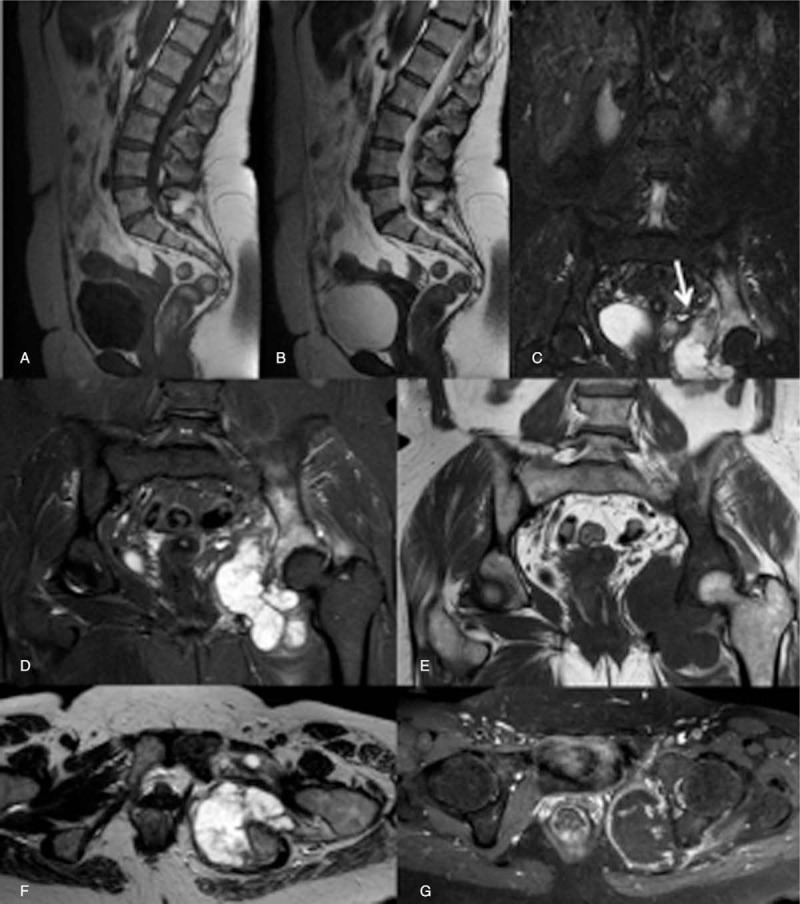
A 52-year-old female patient with chronic low back pain. (A) Sagittal T1-weighted sequence. (B) Sagittal T2-weighted sequence. (C and D) Coronal-STIR sequence. (E) Coronal T2-weighed sequence. (F) Axial T2-weighted sequence. (G) Axial T1-weighted 3D fat-sat MR sequence after contrast agent injection. Conventional MR sequences acquired on sagittal plane showed the presence of a mild disc extrusion at the level of the L5–S1 intersomatic space and a disc protrusion at the level of the L2–L3 intersomatic space (A and B). On coronal-STIR (C), a large expansive mass of the ischiopubic ramus with diffuse signal hyperintensity of the iliac bone was appreciated (arrow). The mass would have been missed on sagittal plane. (D–G) A following MR examination of the ischiopubic region confirmed the presence of a large expansive mass with irregular and peripheral enhancement, suggestive of a bone sarcoma.
